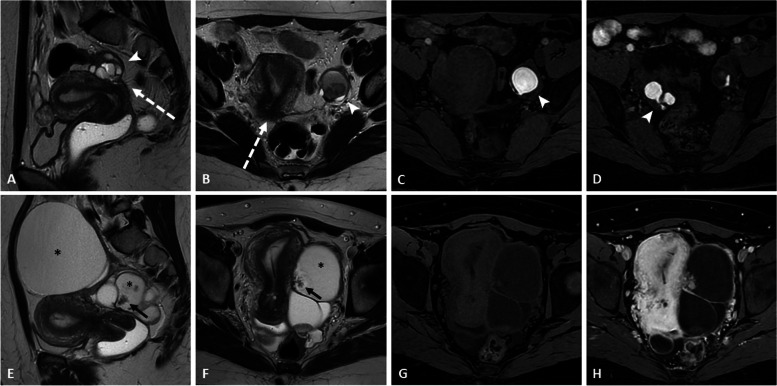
Malignant evolution of an ovarian endometrioma in a 37-year-old woman. Sagittal (a) and axial (b) T2 images and axial fat-suppressed T1 images (c, d) show the pelvic endometriosis with torus uterinum thickening (dotted arrows) and typical bilateral endometriomas (arrowheads). A simple clinical surveillance was decided. MRI scan (axial and sagittal T2 images (e, f) and axial fat-suppressed T1 images before and after injection of gadolinium chelates (g, h)) performed 6 years later due to a palpable abdominal mass showed a large ovarian mixed lesion with a cystic component (asterisk) and endocystic tumoral nodules (black arrow in f). Surgery was performed and found a left ovarian borderline seromucinous carcinoma developed on endometrioma
